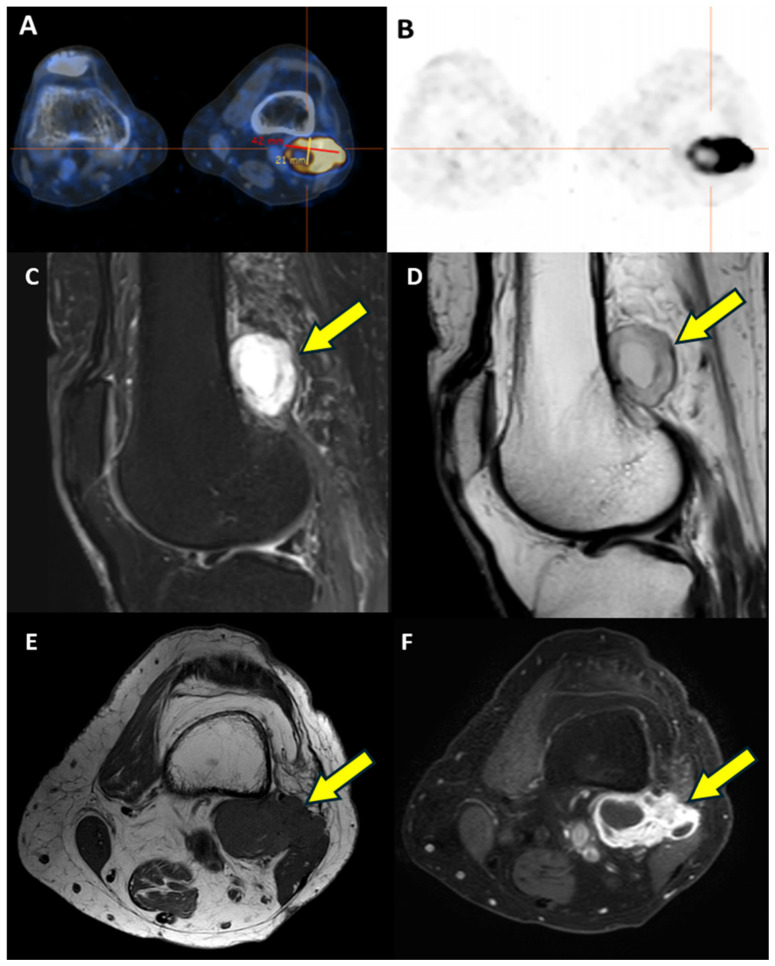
Imaging localization of the phosphaturic lesion. (A,B) ^68^Ga-DOTATOC PET/CT imaging showing intense focal radiopharmaceutical uptake in the left popliteal fossa. (C–F) MRI showed a well-defined multilobulated lesion (yellow arrow) with sharp margins, measuring 22 mm × 43 mm on the axial plane and 30 mm in longitudinal extension, within the soft tissues of the left popliteal fossa, slightly above the left lateral femoral condyle. The lesion appeared heterogeneously hyperintense on sagittal STIR (C) and T2-weighted TSE sequences (D) and hypointense on axial T1-weighted TSE images (E). After gadolinium-based contrast administration, T1-weighted TSE fat-sat images (F) showed intense peripheral and septal enhancement, with a multiloculated appearance and a non-enhancing core. The lesion was adjacent to the femoral cortex, popliteal neurovascular structures, and biceps femoris, without evidence of infiltration or regional lymphadenopathy.
